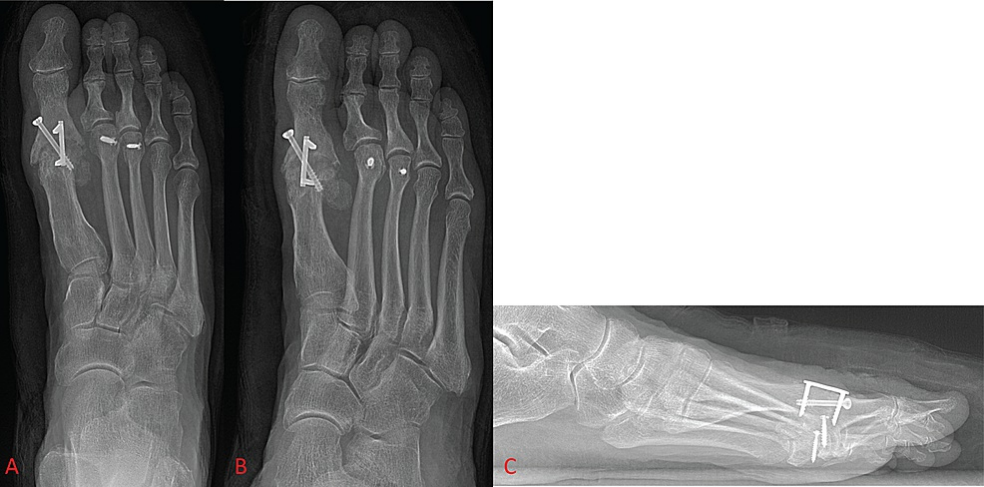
Foot X-ray shows memory compression staple fixation of the first metatarsophalangeal joint with supplementary interfragmentary lag screw fixation. Figure 5A and Figure 5B show anterior-posterior and oblique views of the foot, respectively. Figure 5C shows a lateral view of the foot.
